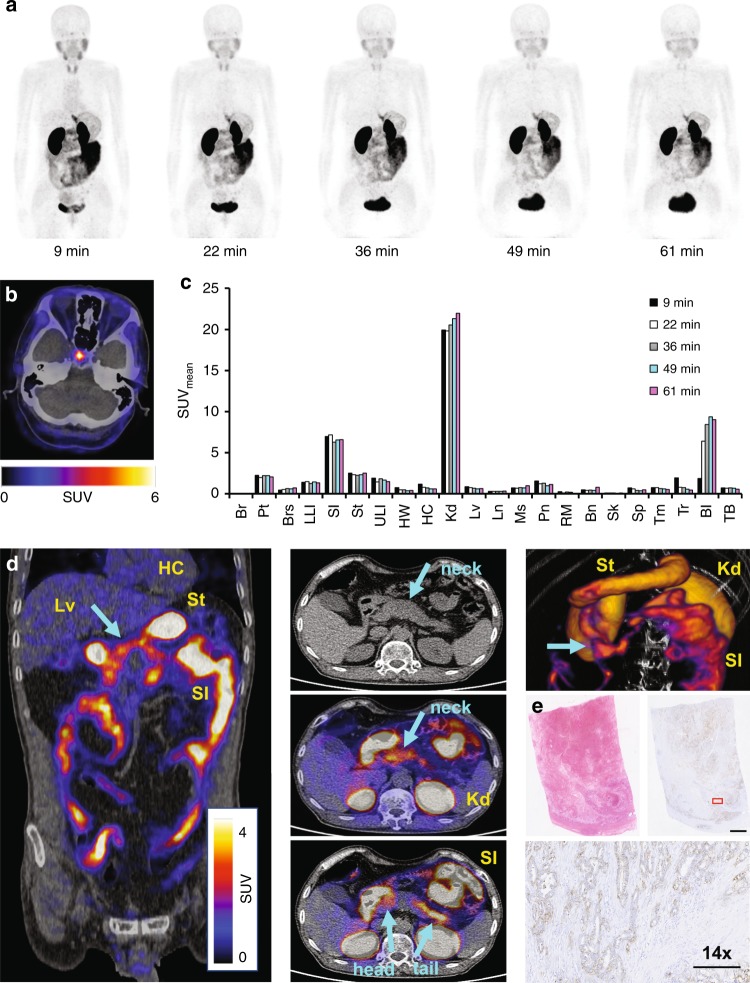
[^68^Ga]NODAGA-R_0_1-MG biodistribution and PET imaging of pancreatic cancer. a Sequential MIP PET images of a 69-year-old female study subject show the biodistribution of the tracer for up to ~1 h after intravenous administration. b PET/CT image of the brain shows focal uptake of the tracer in the pituitary gland. c ROI analysis of the representative patient shown above. The following organs are shown: brain (Br), pituitary gland (Pt), breast (Brs), lower large intestine (LLI), small intestine (SI), stomach (St), upper large intestine (ULI), heart wall (HW), heart contents (HC), kidney (Kd), liver (Lv), lung (Ln), muscle (Ms), red marrow (RM), osteogenic cells (Bn), skin (Sk), spleen (Sp), thymus (Tm), thyroid (Tr), bladder (Bl), and total body (TB). d Coronal and axial PET/CT images (left and center) and volume rendered PET/CT image (top right) acquired from a patient diagnosed with pancreatic cancer. The arrows indicate the location of the tumor. High uptake is observed throughout most of the pancreas including the head (SUV_mean_ ~ 3.1), uncinate process, neck and tail (SUV_mean_ ~ 4.4). Comparatively, the SUV_mean_ = 1.8 ± 0.5 S.D. for normal pancreas (*n* = 5). e IHC confirms integrin α_v_β_6_ expression in the viable part of the tumor. Red box represents area shown at 14x magnification in image below. Pathology report indicated a large amount of necrosis in the tumor. Scale bars on the unmagnified and 14x images represent 2.5cm and 250 mm, respectively. Source data for panel c are provided in a Source Data file
